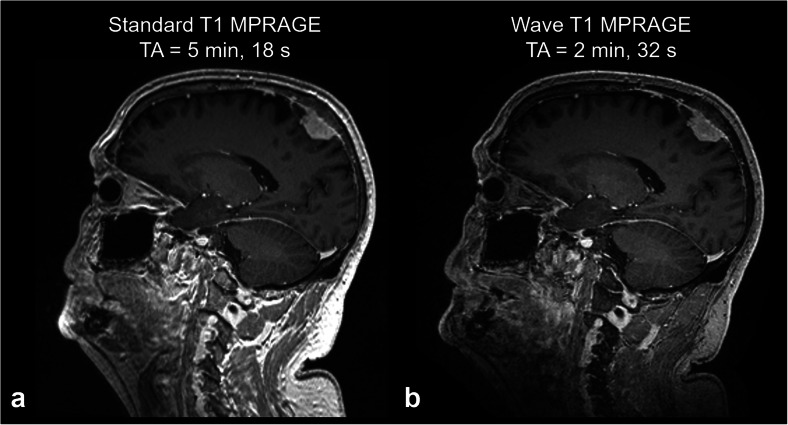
Comparison of post-contrast three-dimensional T1-weighted magnetization prepared rapid acquisition gradient recalled echo (MPRAGE) images acquired with conventional parallel imaging and wave-controlled aliasing in parallel imaging (CAIPI) encoding demonstrating a meningioma. Standard (a) and Wave-CAIPI (b) T1-weighted MPRAGE images show equivalent visualization of the dural-based enhancing mass along the parietal convexity. Both images were acquired on a 3-T Siemens Prisma MRI scanner equipped with 80 mT/m maximum gradient strength and 200 T/m/s maximum slew rate. The Wave-CAIPI sequence was more than twice as fast as the standard sequence (acquisition time = 2 min, 32 s for Wave-CAIPI compared to 5 min, 18 s for the standard sequence)
